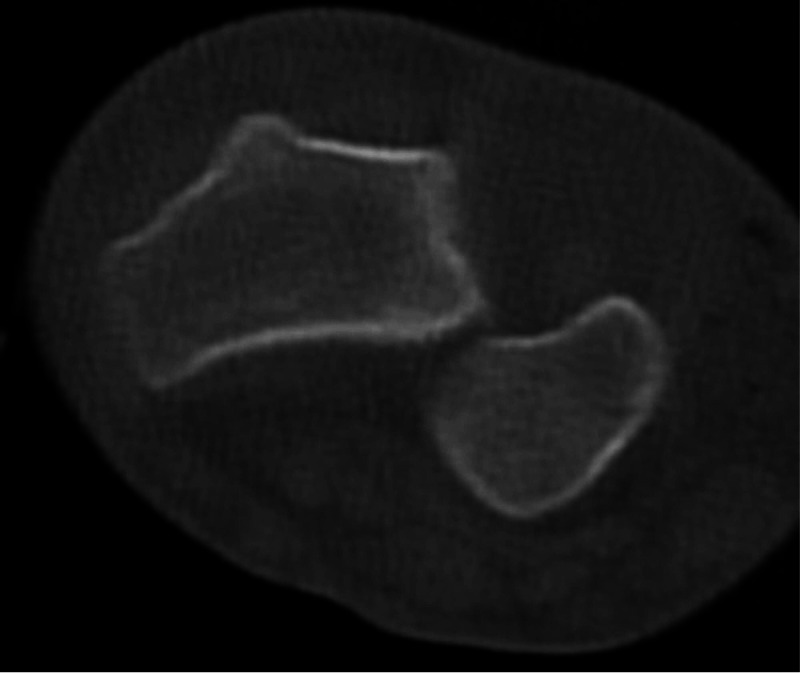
Axial cut CT scan at the level of the distal radioulnar joint CT: computed tomography
